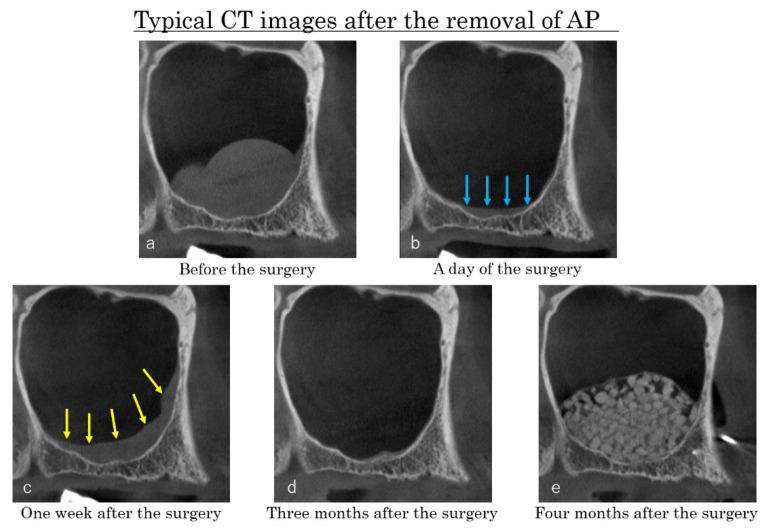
Case: 62 years old female. Two APs were detected on the CBCT before surgery (a). The cysts were removed, and the flat shaped faint opacity at the bottom of maxillary sinus was observed on the CBCT taken immediately after the surgery ((b), blue arrows). One week after the surgery, a post-operative swelling of the sinus membrane was observed ((c), yellow arrows). The swelling had disappeared three months after the surgery (d), and the sinus floor augmentation was performed successfully four months after the removal of Aps (e).
